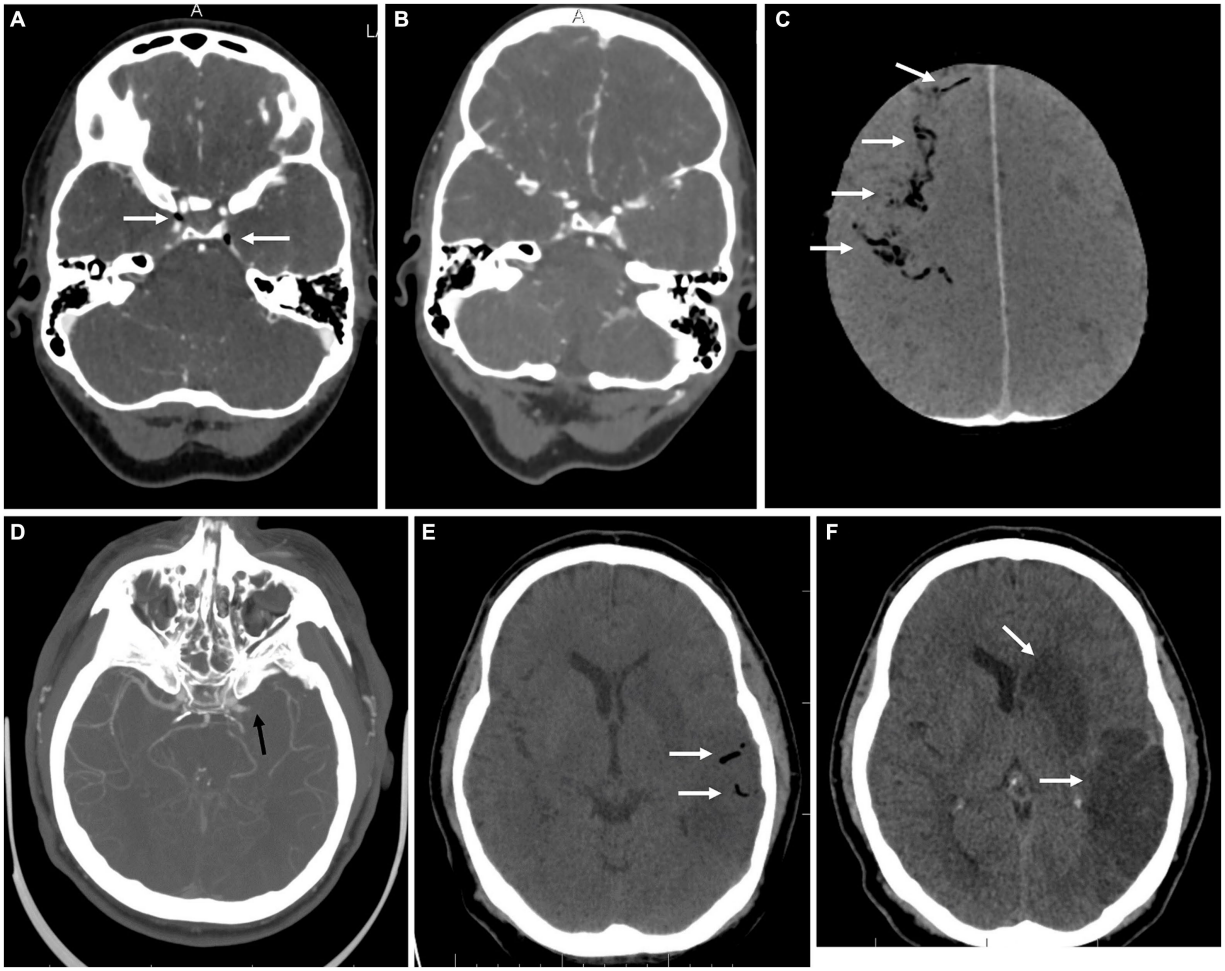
Imaging for case 3. (A) Axial native CT scan of the lungs showing a solid nodulus with an inserted percutaneous biopsy needle (white arrow). (B) Follow-up native CT scan of the lungs after the biopsy with a small pneumothorax and intraparenchymal hemorrhage behind the lesion due to damage to the integrity of the vascularity (white arrow). (C) Axial native CT scan of the brain in MinIP shows a smaller amount of air bubbles in the cerebral circulation (white arrows). (D) Axial contrast-enhanced CT scan of the distal part of the neck with air in the left internal jugular vein (white arrow). (E) Follow-up axial CT scan of the brain in MinIP shows a single residual air bubble in the region of the falx cerebri (white arrow). (F) Axial DWI brain scan showing no diffusion restriction and no evidence of acute ischemia.
